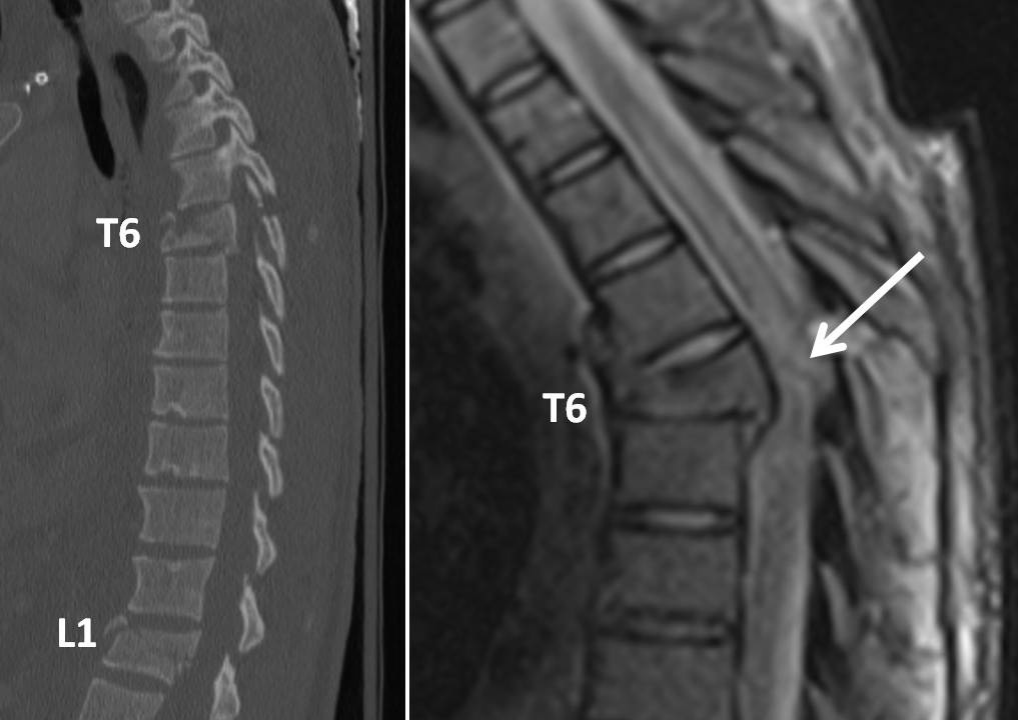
Unstable spine injuries at T6 and L1 on initial multislice CT scan (left panel). The MRI of the T-spine (right panel) revealed a spinal cord transsection at the T6 injury level (arrow).
